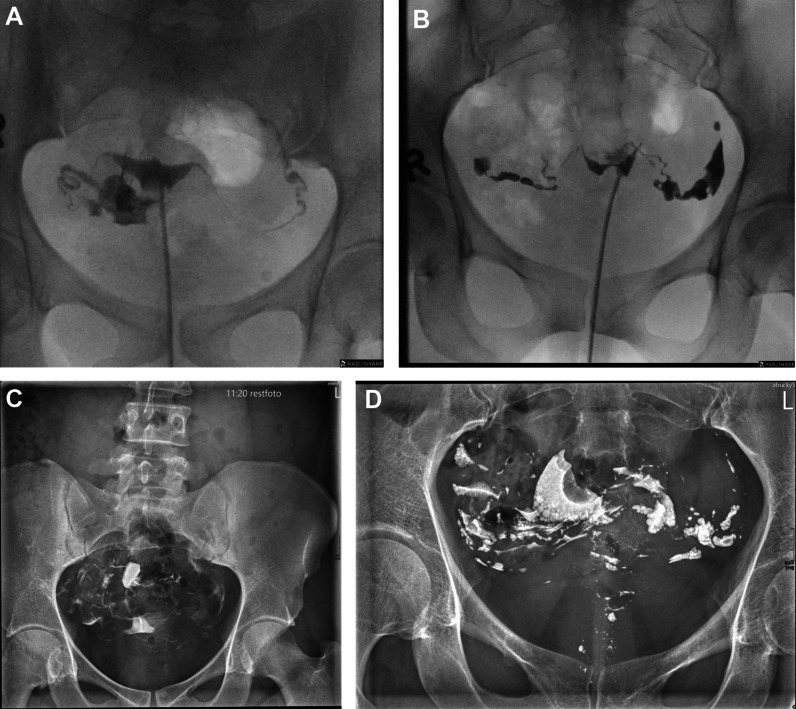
A Normal HSG examinations with symmetric filling of the fallopian tubes and peritoneal spill on both sides: A performed with iodixanol 320, and (B) performed with Lipiodol. Pelvic radiographs obtained 15 min post injection. Note the absence of droplet formation with iodixanol (C) and the presence with  Lipiodol (D). HSG technique: After cleaning of the perineum, a speculum is inserted into the vagina and the cervix is localized and cleaned. A catheter is positioned inside the cervical canal and an occluding balloon is inflated to the extent the patient can tolerate. After a scout radiograph of the pelvis with the catheter in place, contrast material is installed under fluoroscopic guidance and several spot radiographs are taken
